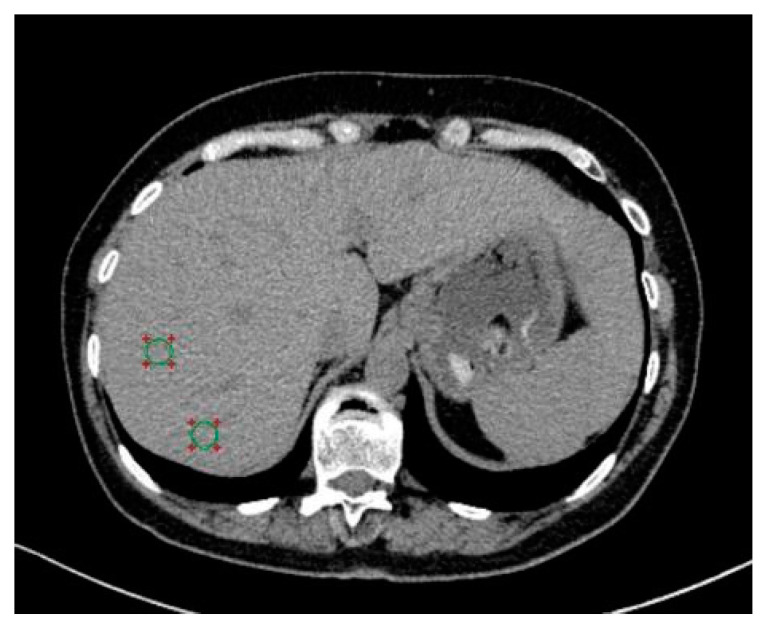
Showing a transverse non-enhanced CT scan with an example of where an ROI should be placed to evaluate attenuation. Two ROIs are positioned in the liver segments VII and VIII, represented by the two green circles.
